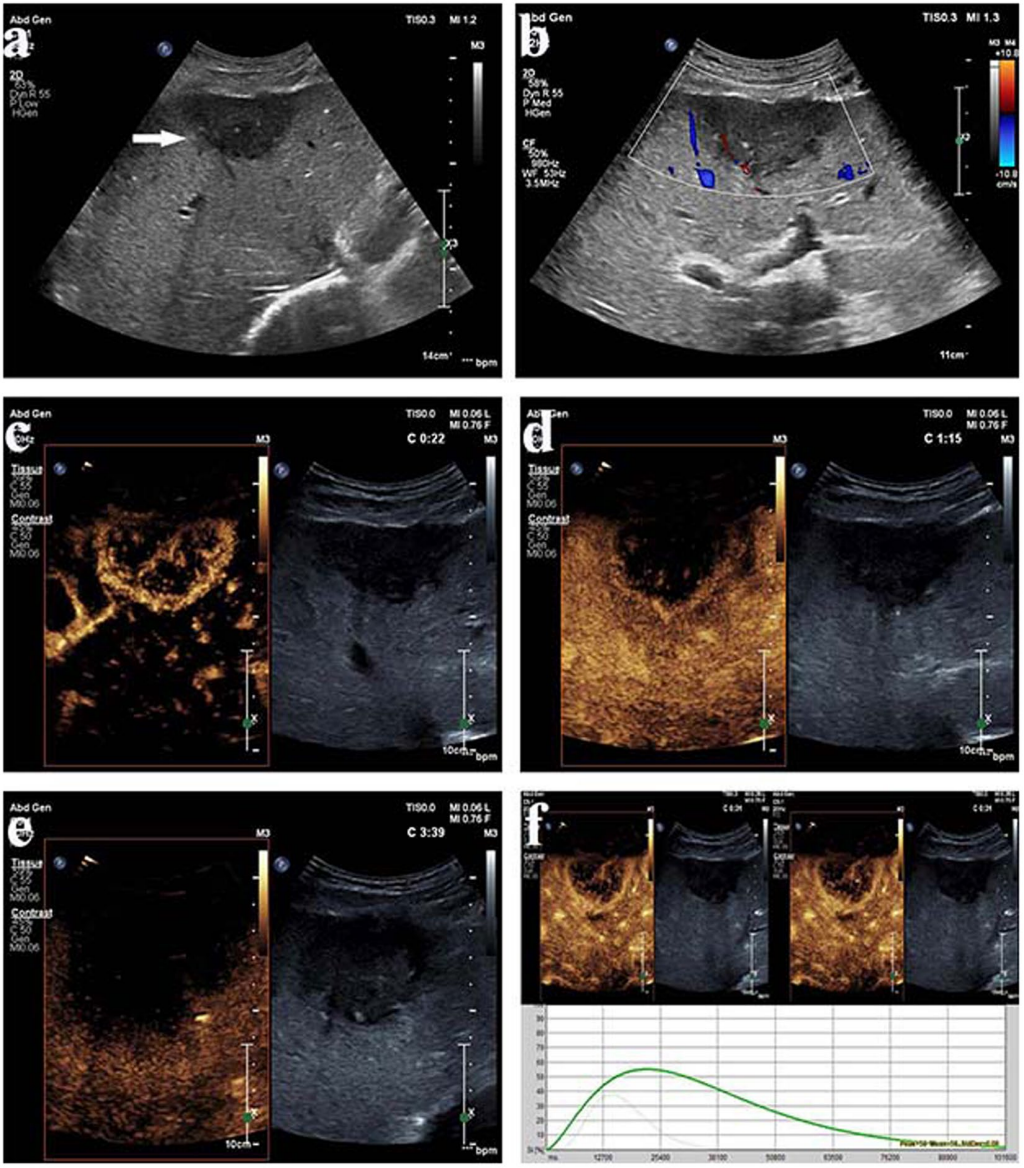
Intrahepatic cholangiocarcinoma (ICC) in a 83–year-old male. (see Supplementary Video S3–4). (a) Conventional ultrasound shows a hypoechoic lesion with a size of 5.5 cm × 3.1 cm in the left lobe of the liver (arrow); (b) Color Doppler sonography indicates a relatively sparse blood supply; (c) During the arterial phase (22 s), the lesion exhibits peripheral rim-like enhancement; (d) During the portal venous phase (75 s), the lesion exhibits hypoenhancement of its interior with hyperenhancement in the peripheral region, resulting in the formation of a hyperenhanced rim; (e) During the late phase (219 s), the lesion and its surrounding area exhibit hypoenhancement; (f) The parametric curves reveal perfusion of the region of interest in the interior (light green, Peak = 38.5, Sharpness = 0.391 1/s) and periphery (dark green, Peak = 56.0, Sharpness = 0.075 1/s) of the lesion from 10 s to 112 s.
